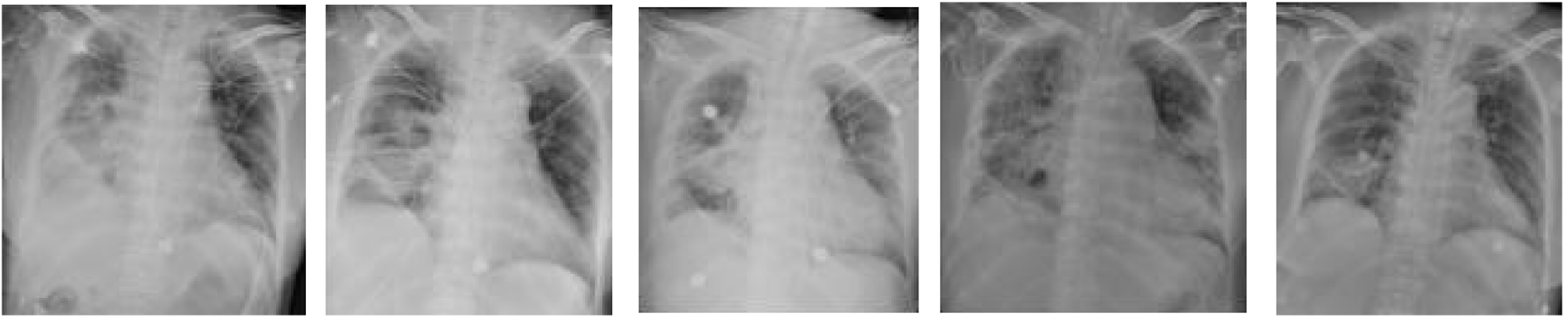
Images of chest x-ray acquired overtime showing changes associated with therapeutic interventions. (A): on arrival, Chest radiograph showed bilateral infectious lung lesions and pleural effusion; (B–D): before the treatment, progressive exudation in both lungs; (E): after treatment, largely resolution was revealed.
